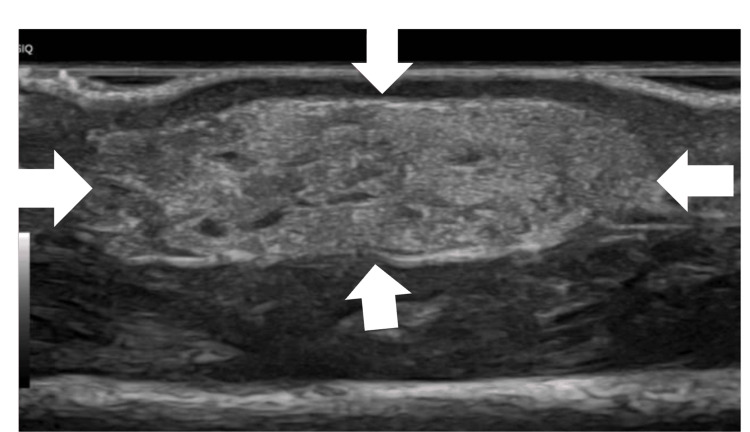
Ultrasound image showing a 14 × 10 × 6 mm hyperechoic, oval lesion, containing small internal cystic components, likely corresponding to a focus of subcutaneous adipose necrosis
